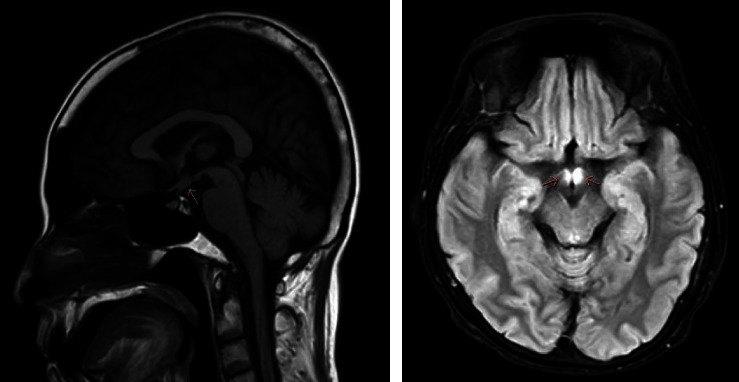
T1 and T2 FLAIR sections of magnetic resonance imaging show hyper signal intensity involving the bilateral hypothalamus.
